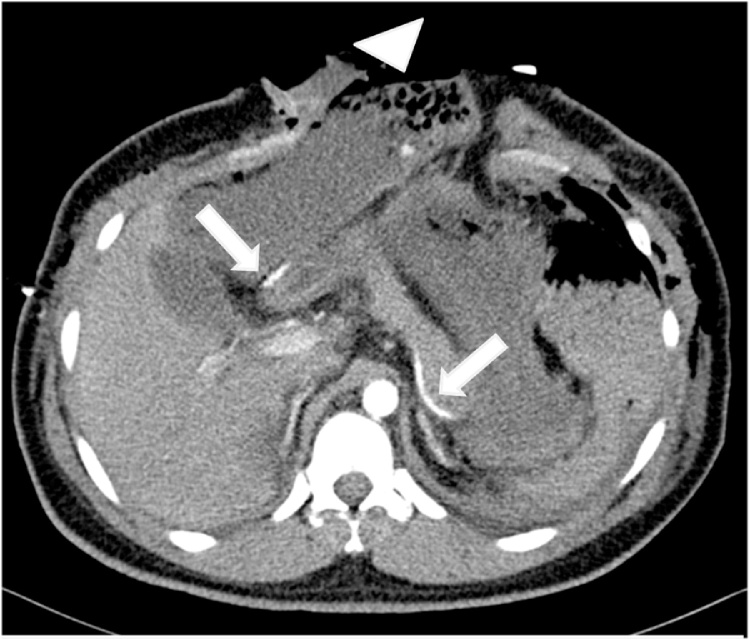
Axial Enhanced CT of the abdomen post explorative laparotomy shows; A: surgical suture lines along the greater curvature of the stomach (bold arrows),Anterior abdominal wall defect with part of the stomach herniating through it (arrowhead).
